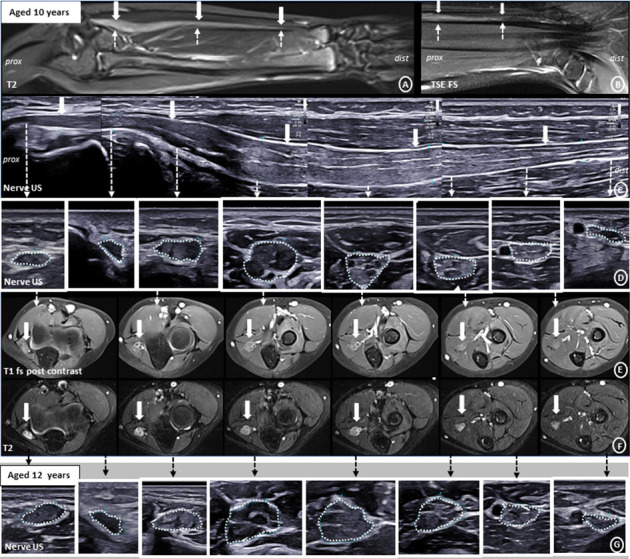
Imaging characteristics of the significant, long-extended enlargement of the right ulnar nerve throughout its entire course from the distal upper to distal lower arm. On coronal T2-weighted MRI (A), coronal Turbo-Spin-Echo fat-suppression MRI (B) and axial T2-weighted MRI (F), a marked hyperintensity of the entire ulnar nerve is visible. Sagittal (C) and axial (D) high-resolution nerve ultrasound depicts the pronounced fusiform enlargement of the entire nerve in the forearm, with multifascicular involvement, predominantly hypoechogenic, but partly inhomogeneous with hyperechogenic components. See also Videos 1–5 (Supporting Information) for a detailed documentation. Markedly reduced fascicular differentiation (“blurry” appearance) of the ulnar nerve in the proximal forearm (D) which becomes more pronounced in the follow-up after 2 years (G). Signs of contrast enhancement, especially in the perineurium, on axial T1-weighted MRI (E).
